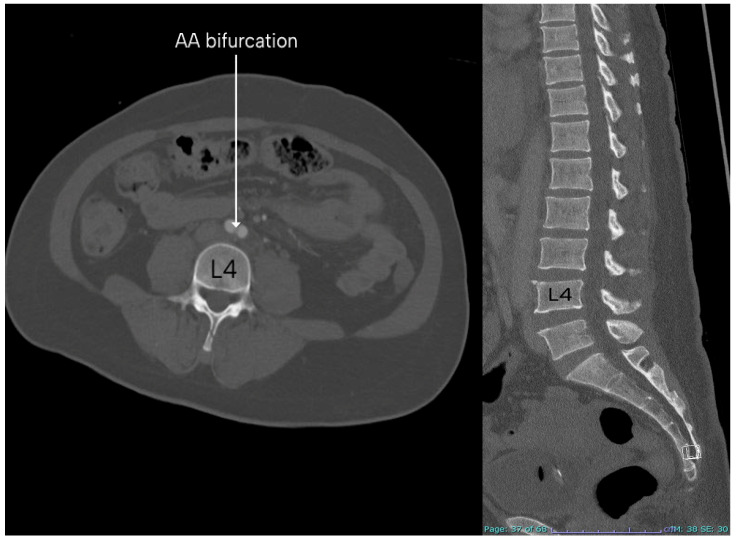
Two CT sections of the same patient. The axial section shows the bifurcation of the AA, and the vertebral level can be observed in the sagittal section. AA: abdominal aorta.
